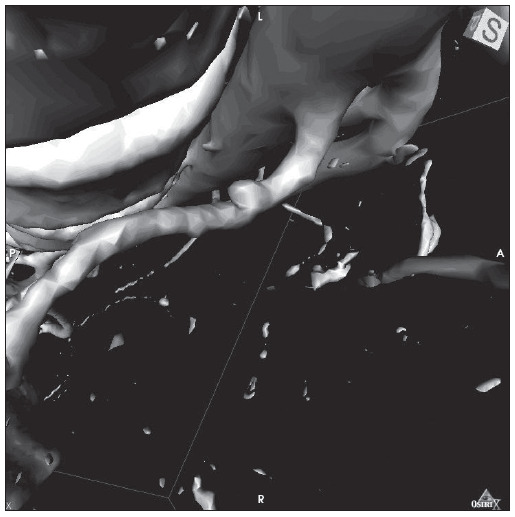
Three-dimensional reconstruction from computed tomography of Case 3: left renal artery pseudoaneurysm.
